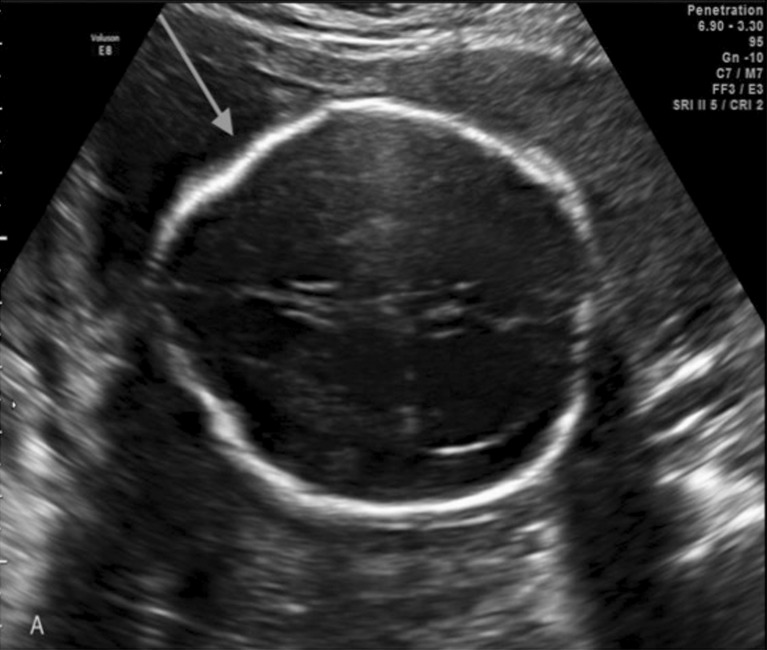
Two-dimensional ultrasonography. The “lemon sign”—the skull in a shape of a lemon due frontal scalloping bone caused by intracranial hypotension
